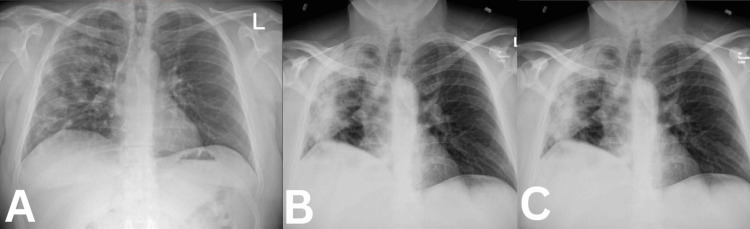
Chest X-rays Chest X-ray demonstrated extensive consolidation in the right lung, suggestive of pneumonia (Figure 1 A) while repeat chest X-rays revealed areas of consolidation in the right lung and apex with associated atelectasis (Figures 1 B, 1C).
